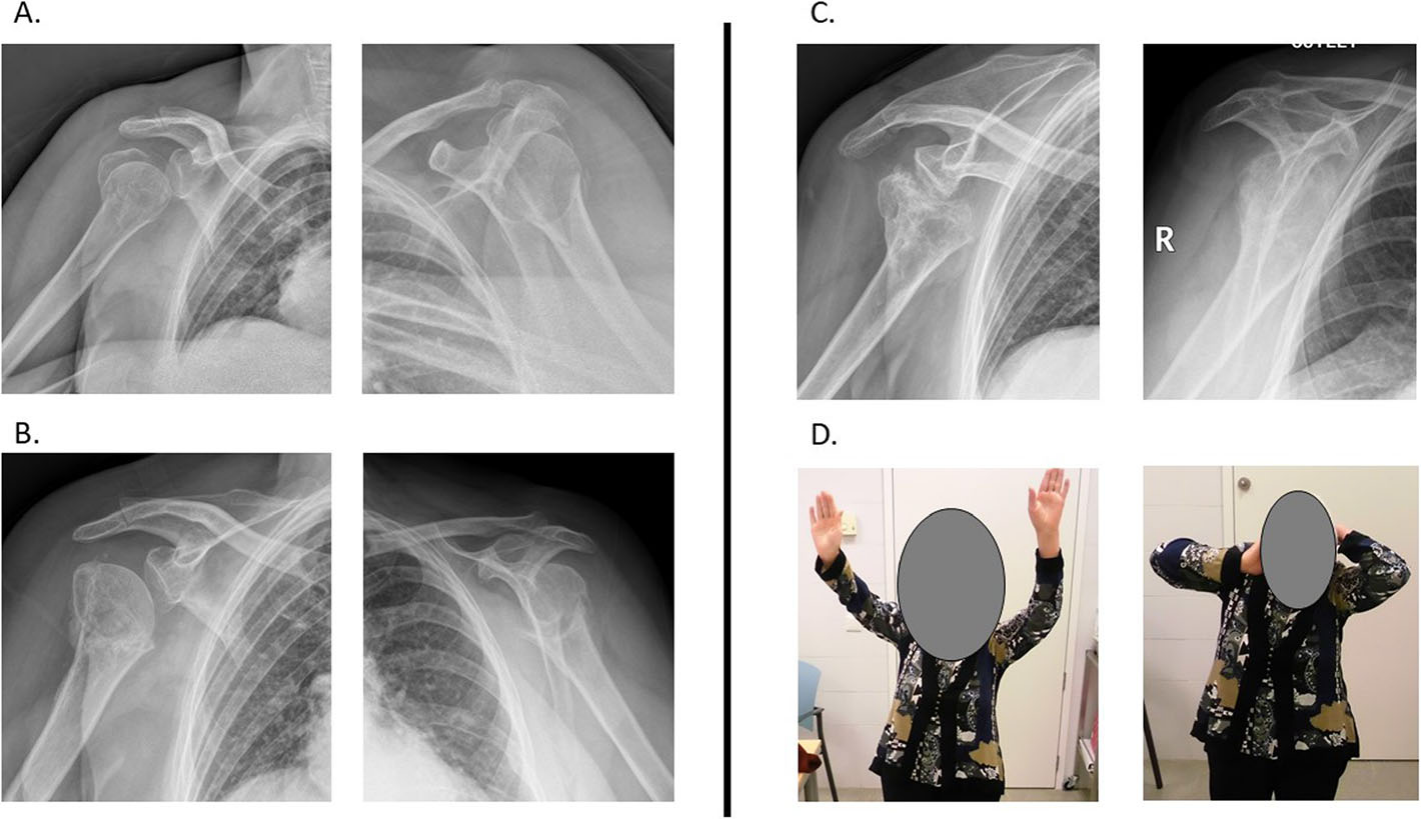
A Initial X-ray presentation of a 61-year-old female with a proximal humeral fracture. B Radiological results at the 6-month follow-up. C Radiological results at the 3-year follow-up. D Clinical results at the 3-year follow-up with a Constant-Murley score of 49 points
